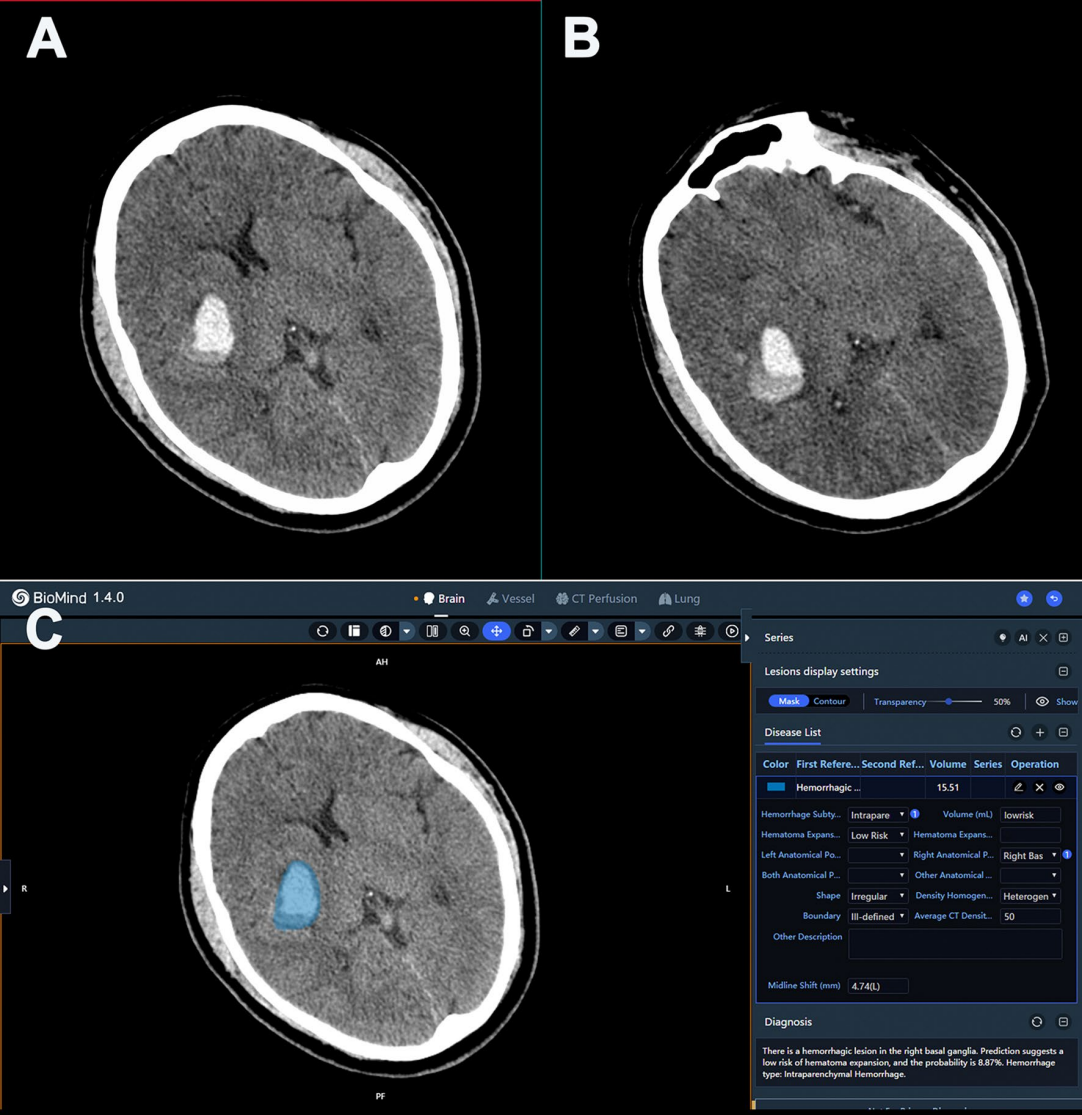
A Initial CT scan image of a 38-years-old male HICH patient. The patient was diagnosed with hematoma expansion by doctors based on CT signs. B Follow-up CT scan within 12 h after the initial scan. The hematoma volume increased by 2.4 ml and was confirmed by the neuroradiologist as non-hematoma expansion. C AI software automatically analyzed the initial CT scan images for hematoma expansion. The patient was diagnosed without hematoma expansion
